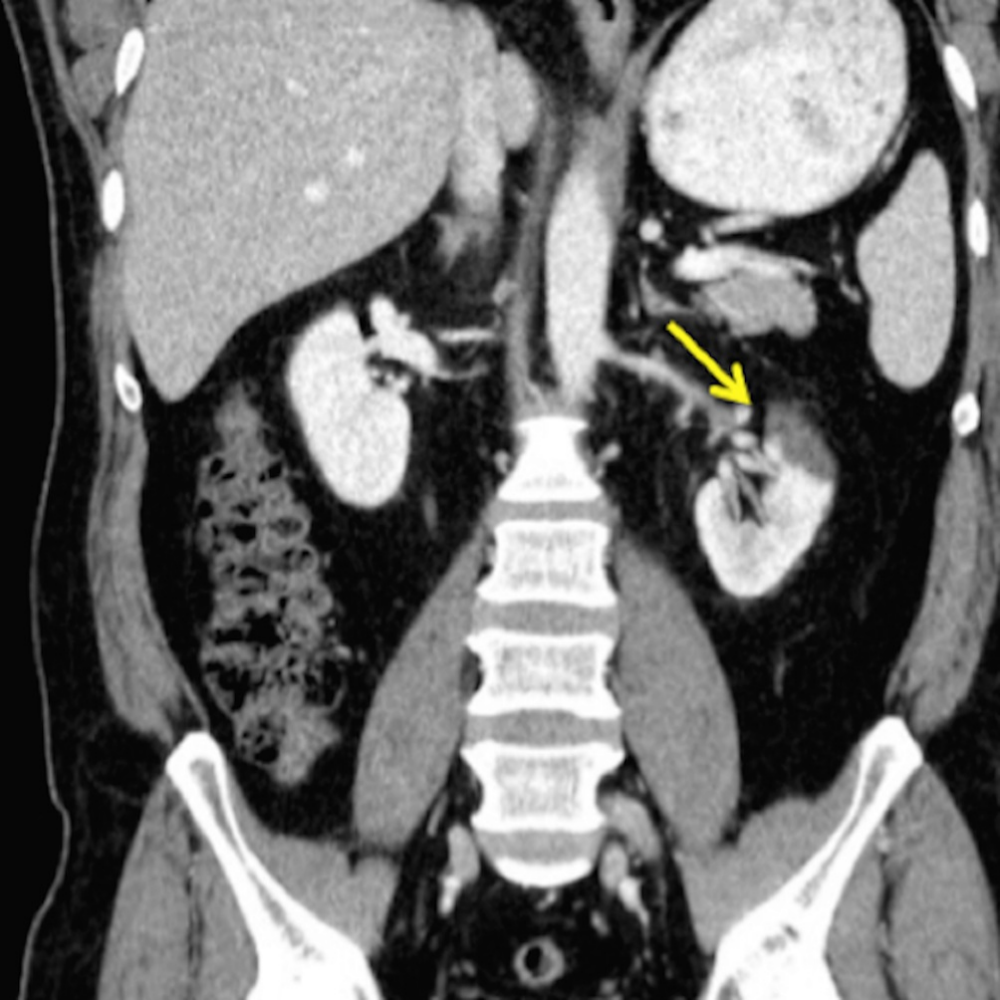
CT scan of abdomen and pelvis - poor opacification of the upper segment of the left kidney, suggestive of infarction (arrow) CT - computed tomography
